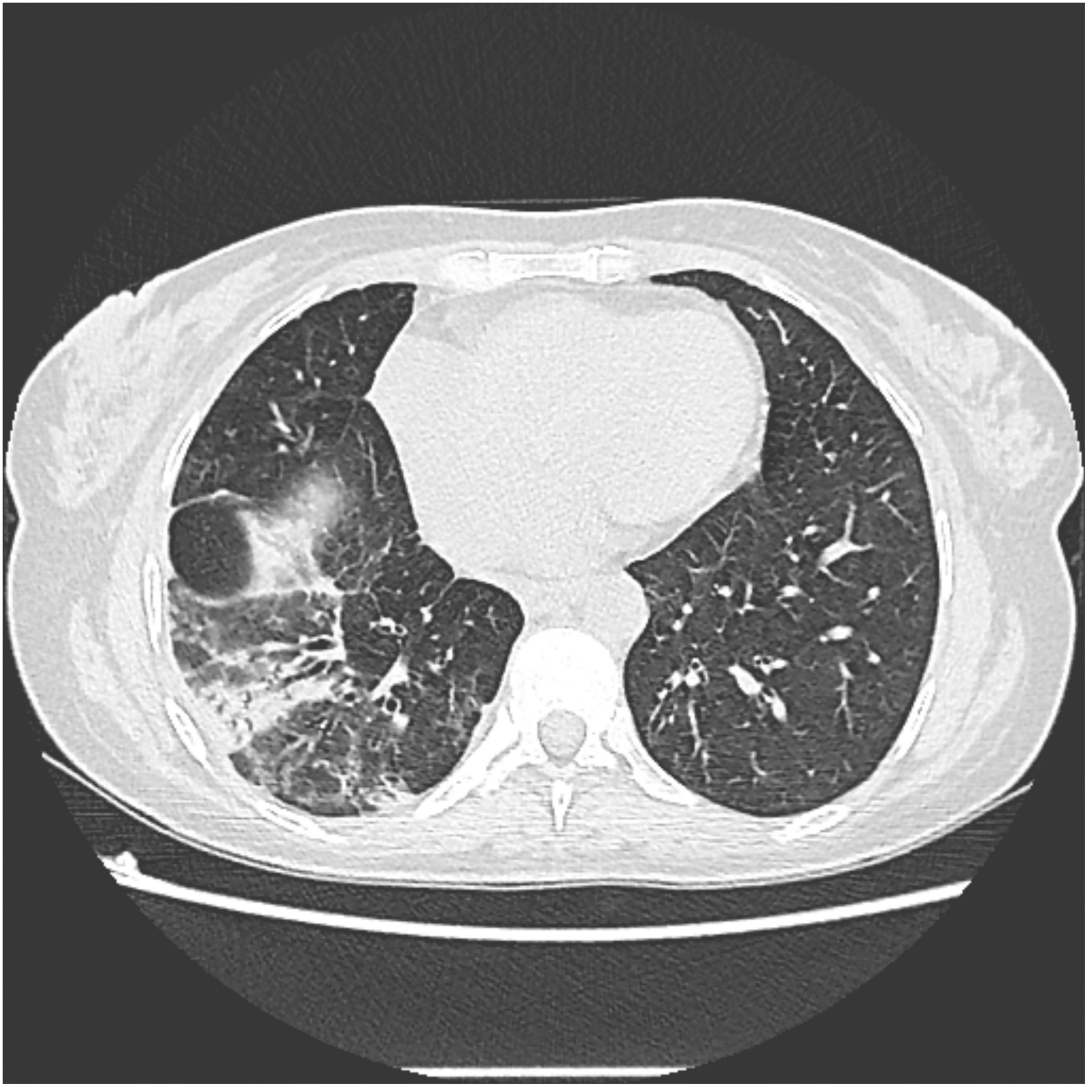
Slice of CT scan showing parenchymal fibrosis and bronchiectasis in the right lower lobe.
